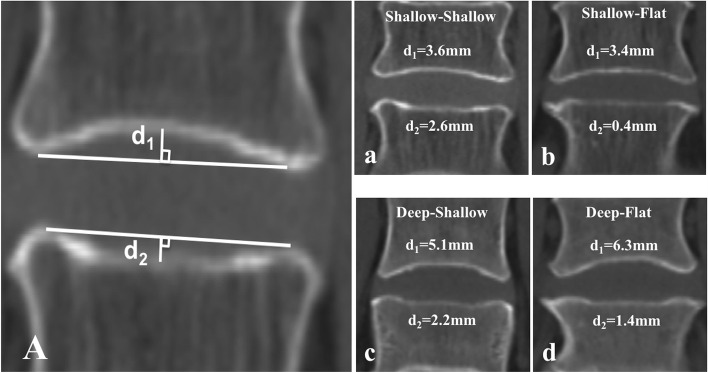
A) Endplate concavity depth (ECD) measured by coronal CT imaging (the level corresponds to the mid-sagittal image) from the concavity apex to the line connecting the left and right apex of the endplate. d_1_ and d_2_ represent the ECD of L4 IEP and L5 SEP respectively. (a-d) The four types of L4-5 intervertebral space (IVS)
